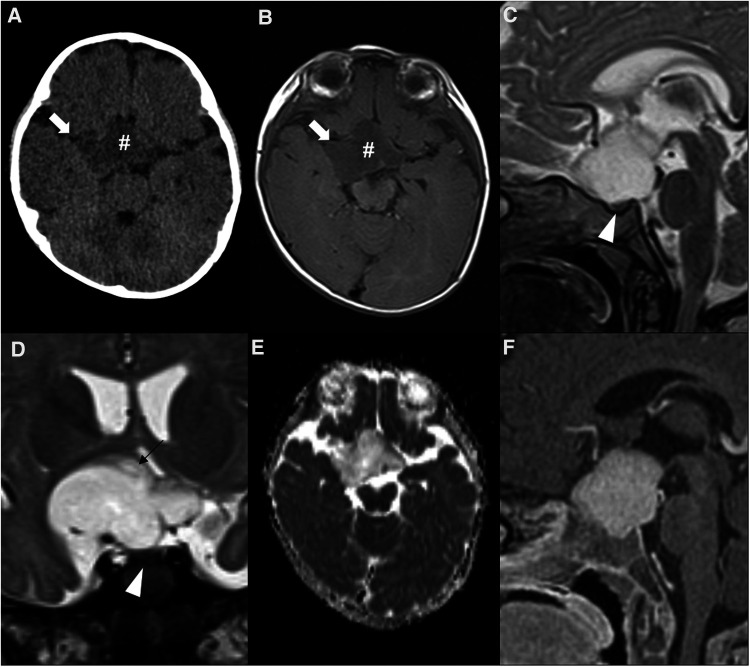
Sporadic hypothalamic and optic pathway astrocytoma in a 7-month-old girl presenting with horizontal nystagmus. Axial head CT (A) and axial brain MRI T1WI (B) shows a hypodense and hypointense expansile lesion (hashtags) centered in the suprasellar region, with extension towards the sylvian fissures, mainly on the right (white arrows). Sagittal (C) and coronal (D) T2WI show a T2 hyperintense lesion which is separated from the pituitary gland (white arrowheads) and originating posterior from the optic chiasm, most probably from the right hypothalamus (black arrow in D). The chiasm and optic tracts (right optic tract is pointed out by the black arrowhead in D) are splayed but not overtly infiltrated. There are no optic nerve changes (not shown). Facilitated diffusion is seen on ADC map (E), as well as homogeneous contrast enhancement on sagittal T1WI after gadolinium injection (F).
